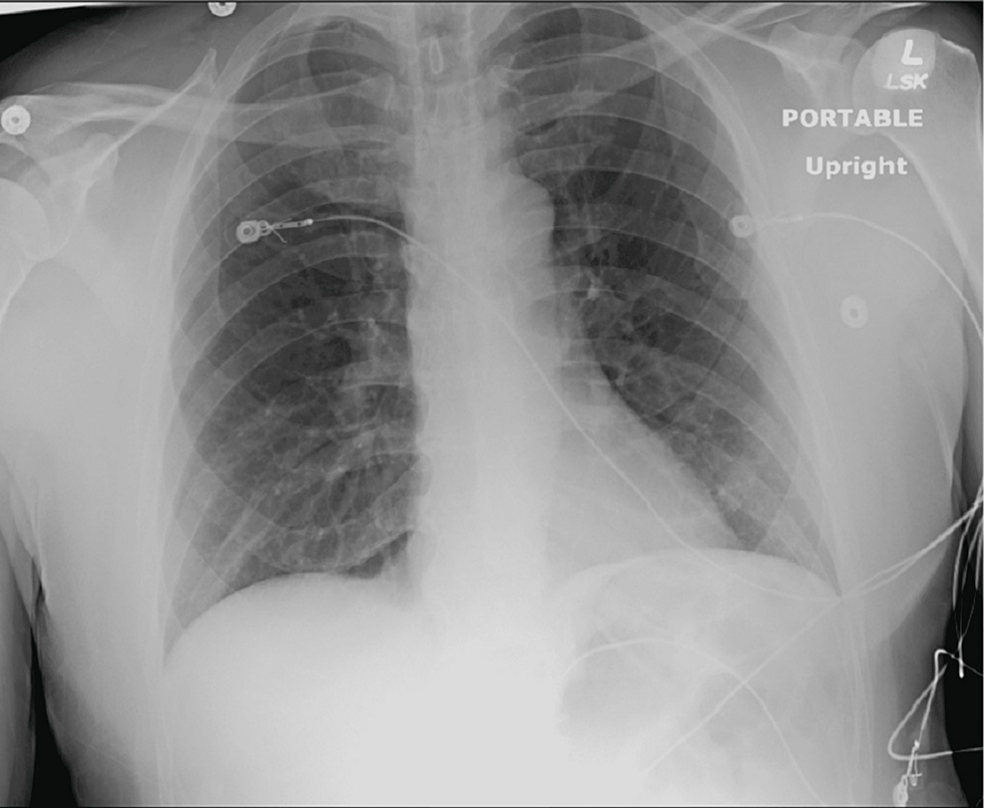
Chest x-ray showing no lymphadenopathy or obvious cardiopulmonary pathology
